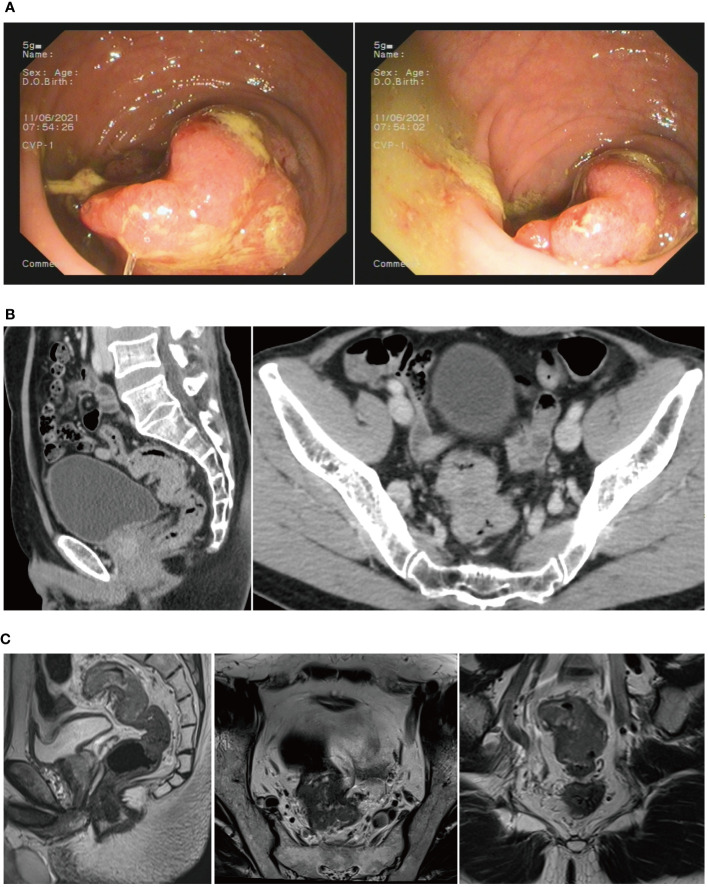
(A) Colonoscopy revealed a rectal cauliflower-like neoplasm. (B) CT scans showed a tumor-like lesion in the rectum. (C) MRI suggested rectal cancer.
